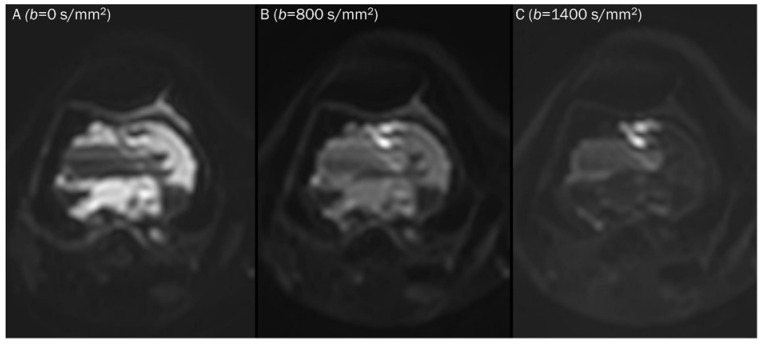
Progressive increase in *b*-values (same patient in Figure 1). As *b*-values increase on DWI (A–C), the perfusion effect is gradually suppressed and only hypercellular tissues remain bright at high *b*-value images with a darkening background.
